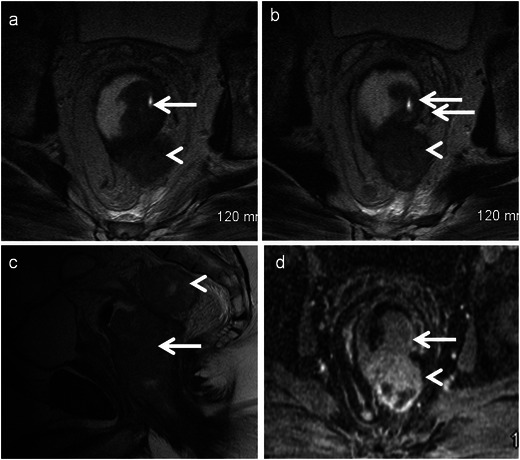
Primary amelanotic anorectal melanoma. a, b Axial T2-weighted MRI reveals fungating intraluminal mass (*arrow*) with a large perirectal deposit (*arrowhead*). c Sagittal T2-weighted MRI demonstrates the polypoidal mass (*arrow*) expanding the rectum and the large perirectal deposit (*arrowhead*). d Axial T1-weighted post contrast MRI reveals heterogeneous enhancement of the mass (*arrow*) and the deposit (*arrowhead*)
